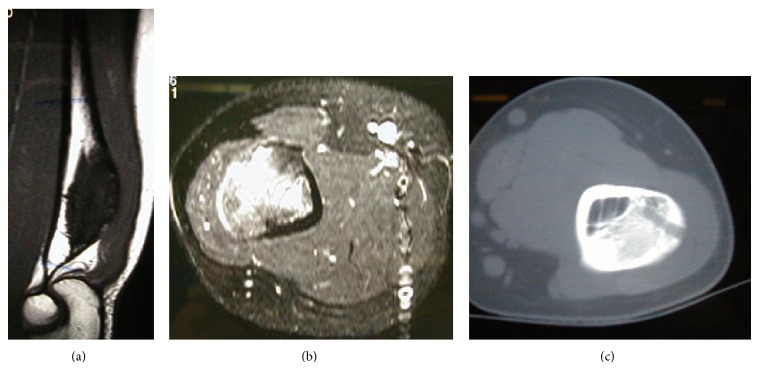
The MRI images show an exostosis arising from the posterior surface of the distal humerus. This lesion measured approximately 6 cm in its long axis terminating immediately proximally to the level of the olecranon and with involvement of the underlying medulla. You can see the sign of the biopsy (c).
